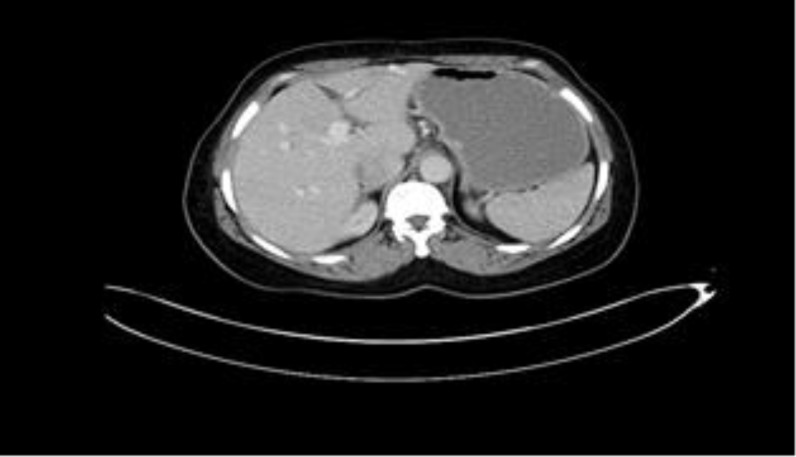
Postoperative computed tomography after six years
